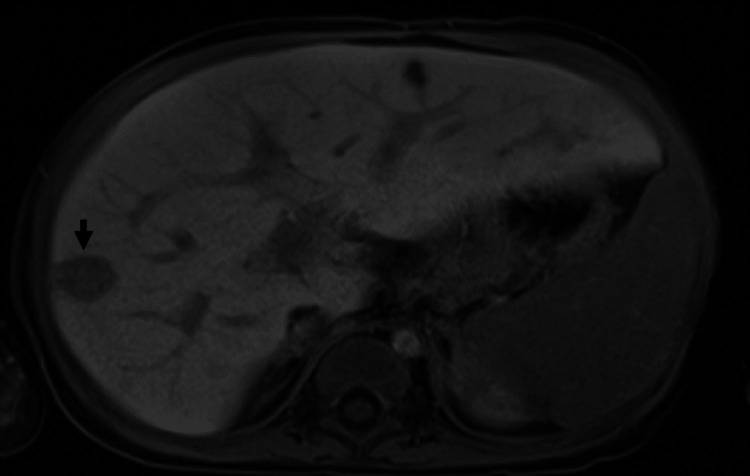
Representative section of a magnetic resonance imaging scan of the liver The largest hepatic lesion, measuring 1.6 x 1.4 cm, is indicated by the black arrow.
